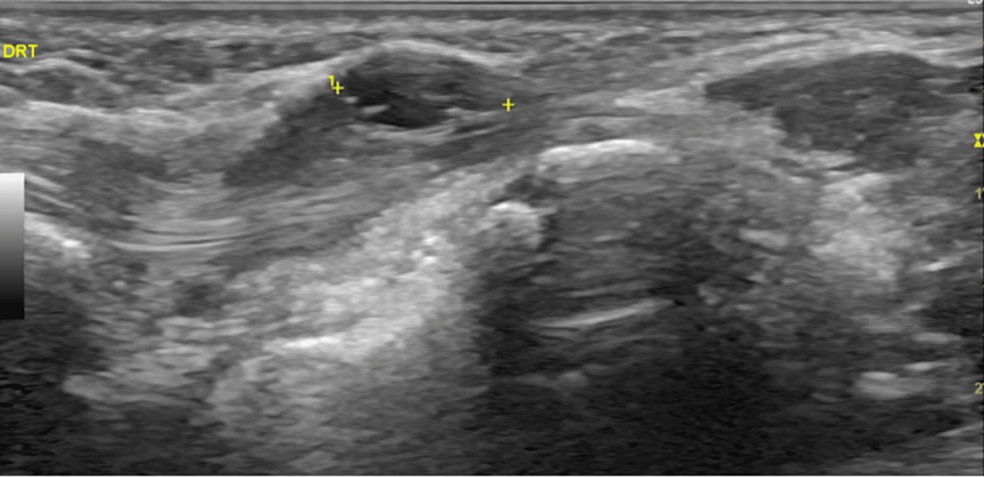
Ultrasound of intratendinous ganglio cyst at the extensor indicis
